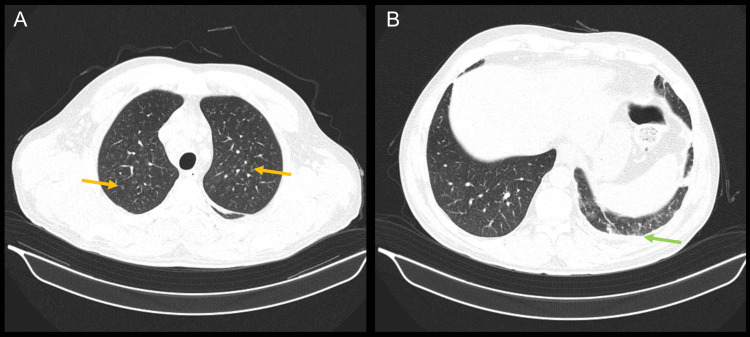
Thoraco-abdominal-pelvic CT scan: lung window (A) Diffuse ground-glass peribronchovascular densifications (yellow arrows). (B) Left pleural effusion (green arrow).
